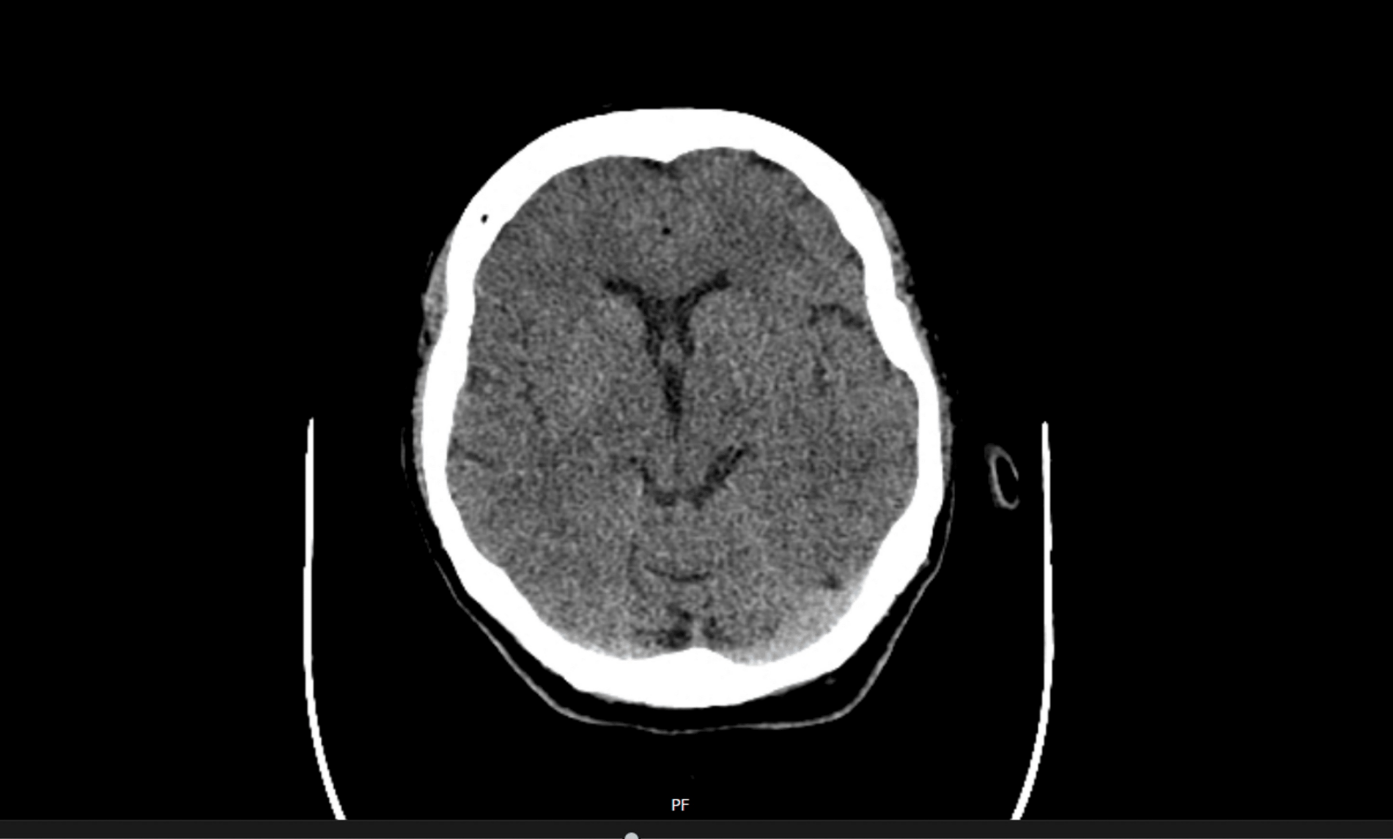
CT head demonstrating normal brain parenchyma CT: Computed tomography.
